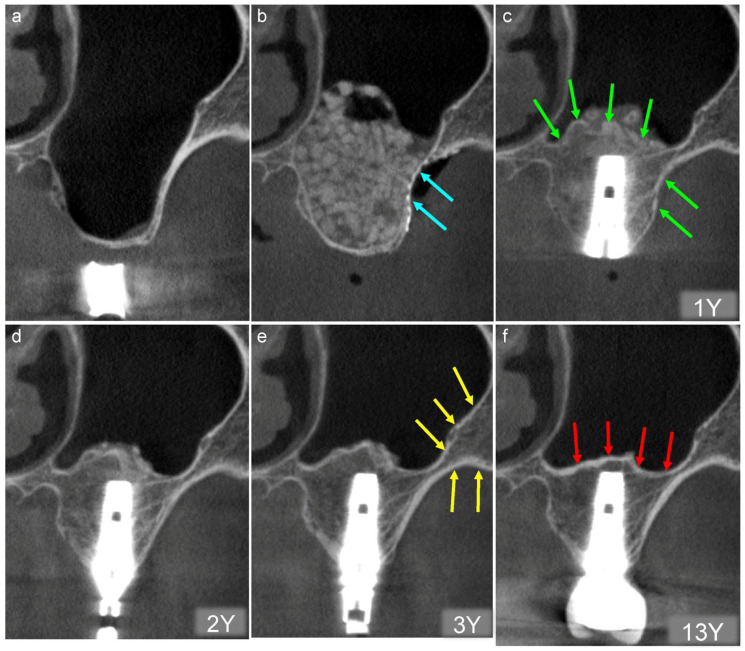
(a) Buccolingual section of the CT image obtained before sinus floor elevation. Bone height is <1 mm. (b) CT image acquired on the day of the surgery. The β augmented area is filled with TCP granules, and the bony window is covered tightly with a titanium mesh plate and microscrews (blue arrows). (c) Tapered-type implants were placed one year postoperatively, and initial fixation was achieved. A clear white line and radiopaque line resembling the cortical bone can be observed at the newly formed bottom of the maxillary sinus and bony window, respectively (green arrows). 1Y; one year follow-up (d) The radiopacity of the augmented area is divided into two types two years postoperatively: cortical and cancerous. 2Y; two years follow-up (e) The radiopacity of the augmented area shows a density similar to that of the original bone (yellow arrows) three years postoperatively. 3Y; three years follow-up (f) The bottom line of the maxillary sinus decreased slightly because of pneumatization 13 years postoperatively (red arrows). 13Y; thirteen years follow-up.
